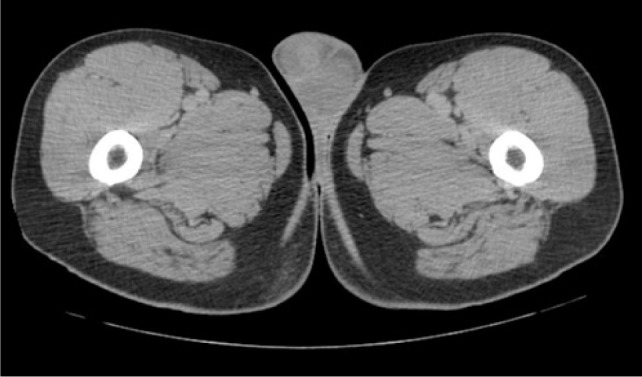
CT urogram showing 1.7-cm enhancing mass in the lower pole of right testicle.
